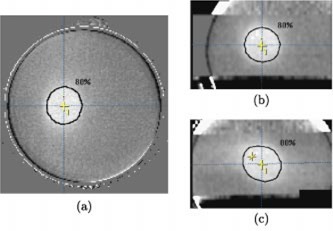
(Color) (a) Axial, (b) coronal, and (c) sagittal CT images of PAG1 (irradiated to a maximum dose of 15 Gy) at isocenter. The coronal and sagittal images were reconstructed from the set of axial gel images (3 mm slice spacing). The planned 80% isodose contour and a cross indicating the isocenter are marked in each image.
